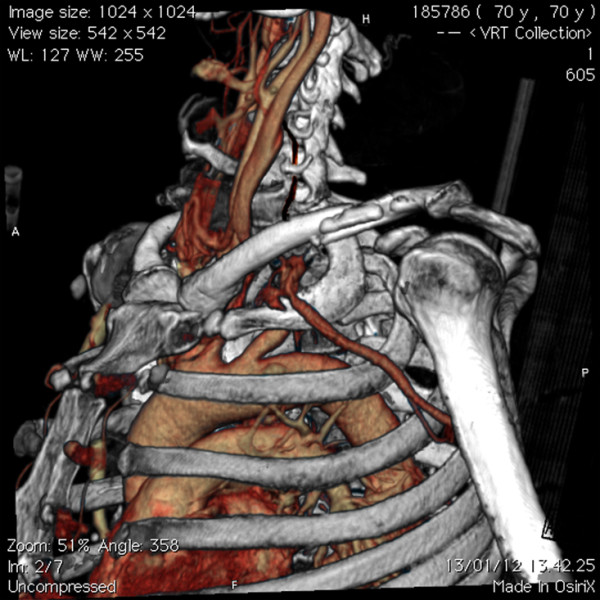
CT 3D reconstruction showing active left subclavian arterial bleeding and the left midshaft clavicular fracture.
